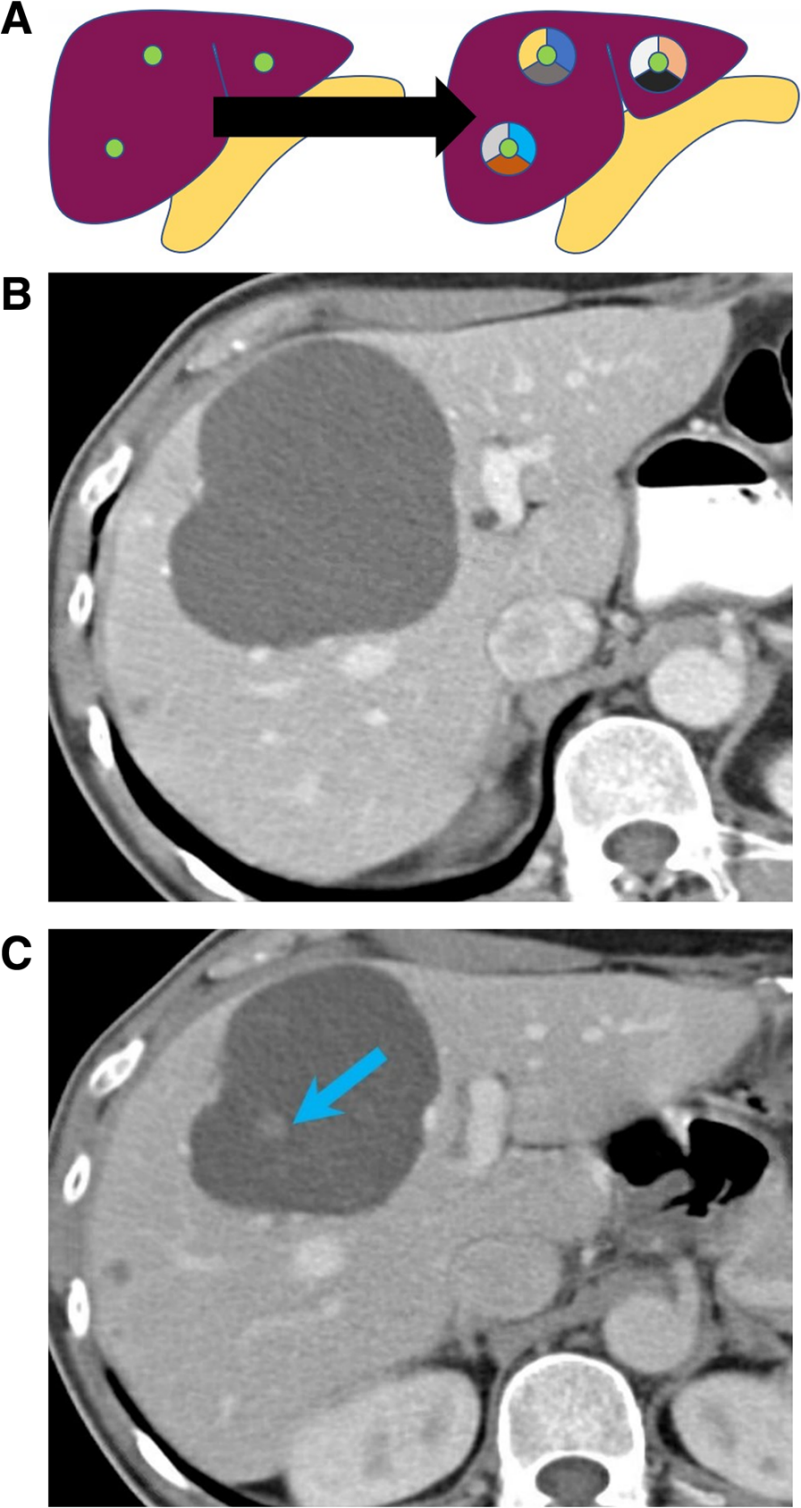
Intrametastatic heterogeneity. a Image exemplifying the development of heterogeneity among the cells the liver metastases as the metastases grow (black arrow). Axial CT images acquired during portal venous phase (b, c) in a 77-year-old woman with gastrointestinal stromal tumor acquired during treatment with imatinib. The hypodense metastasis in the liver shows a new intralesional soft tissue nodule at follow up scan (arrow) (c), suspicious for recurrence
